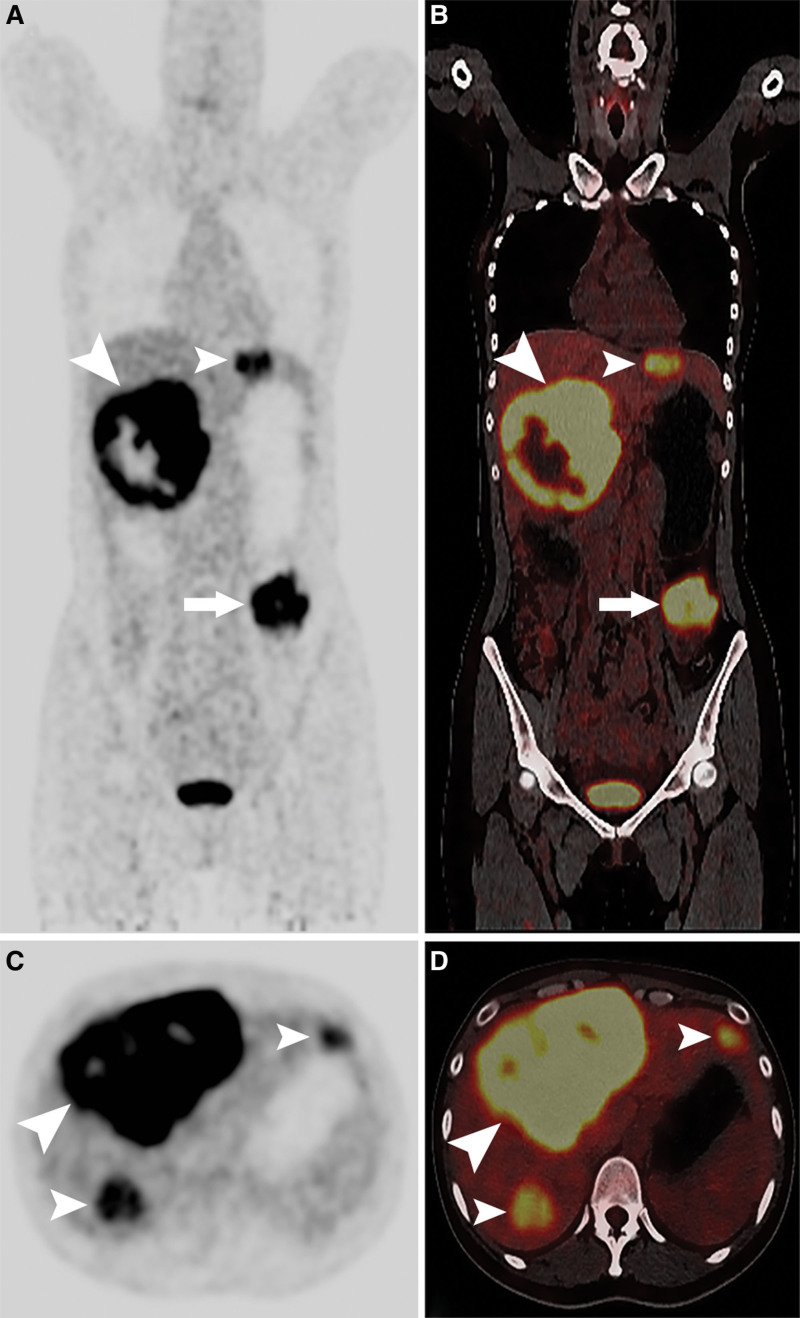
18F-FDG PET/CT images in a case of primary hepatic YST (arrowheads) with intra-abdominal metastasis (arrows). (A) maximum intensity projection 18F-FDG PET; (B) fused PET/CT image; (C) axial PET image; (D) axial fused PET/CT image. ^18^F-FDG = fluorine-18 fluorodeoxyglucose, CT = computed tomography, PET = positron emission tomography, YST = yolk sac tumor.
